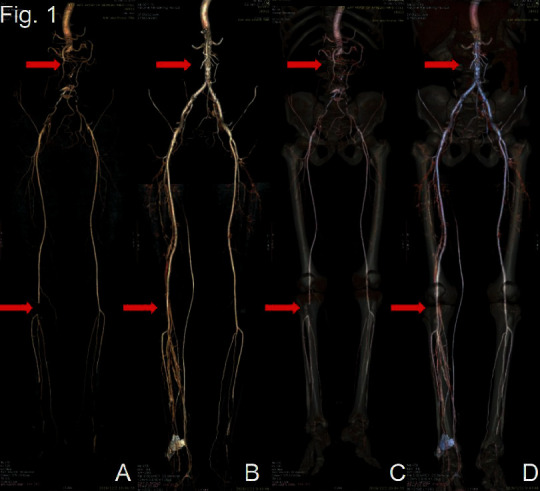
CTA before operation ((a) and (c)) after the operation ((b) and (d)). The red arrow points to the location of the lesion before and after the operation.
